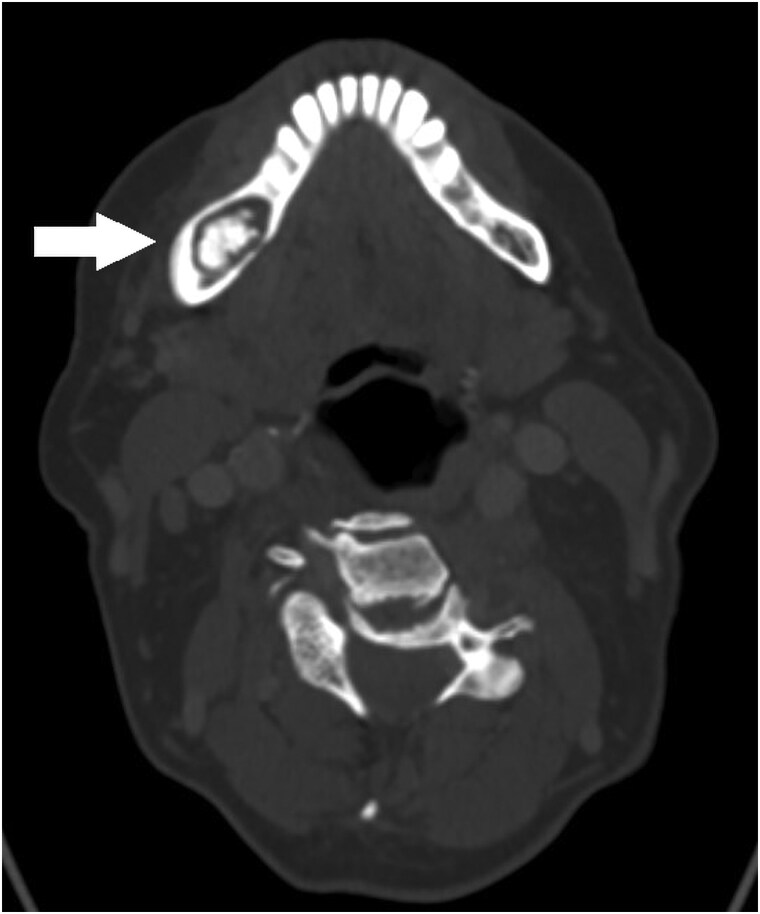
Computed tomography scan (axial view) from 2023 showing an intraosseous lesion of the right mandible. Image courtesy of the Department of Radiology, Nordland Hospital Trust.
